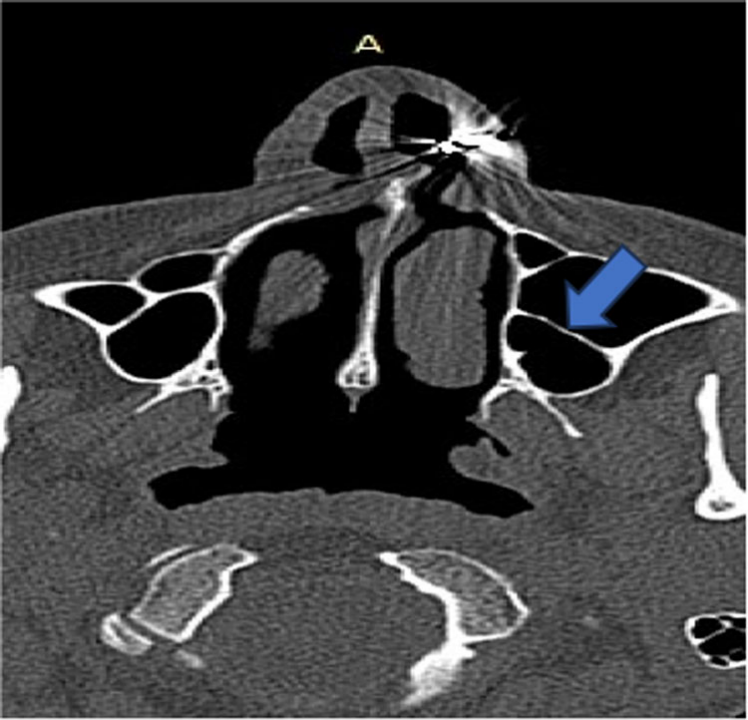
CT axial image shows septations of maxillary sinus.
